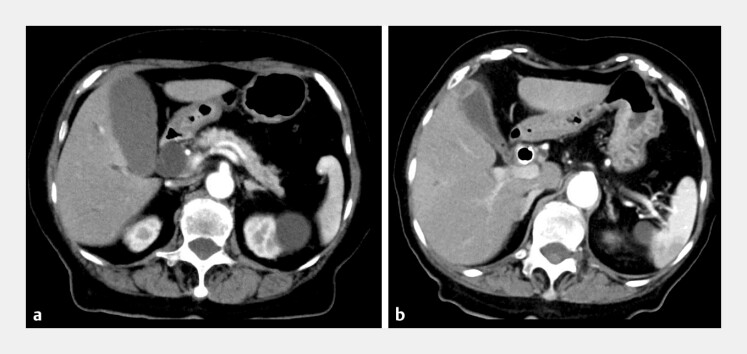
Case 2: Computed tomography showed the gallbladder collapse compared with before placement of the novel HILZO stent (ABIS Inc., Hyogo, Japan).
